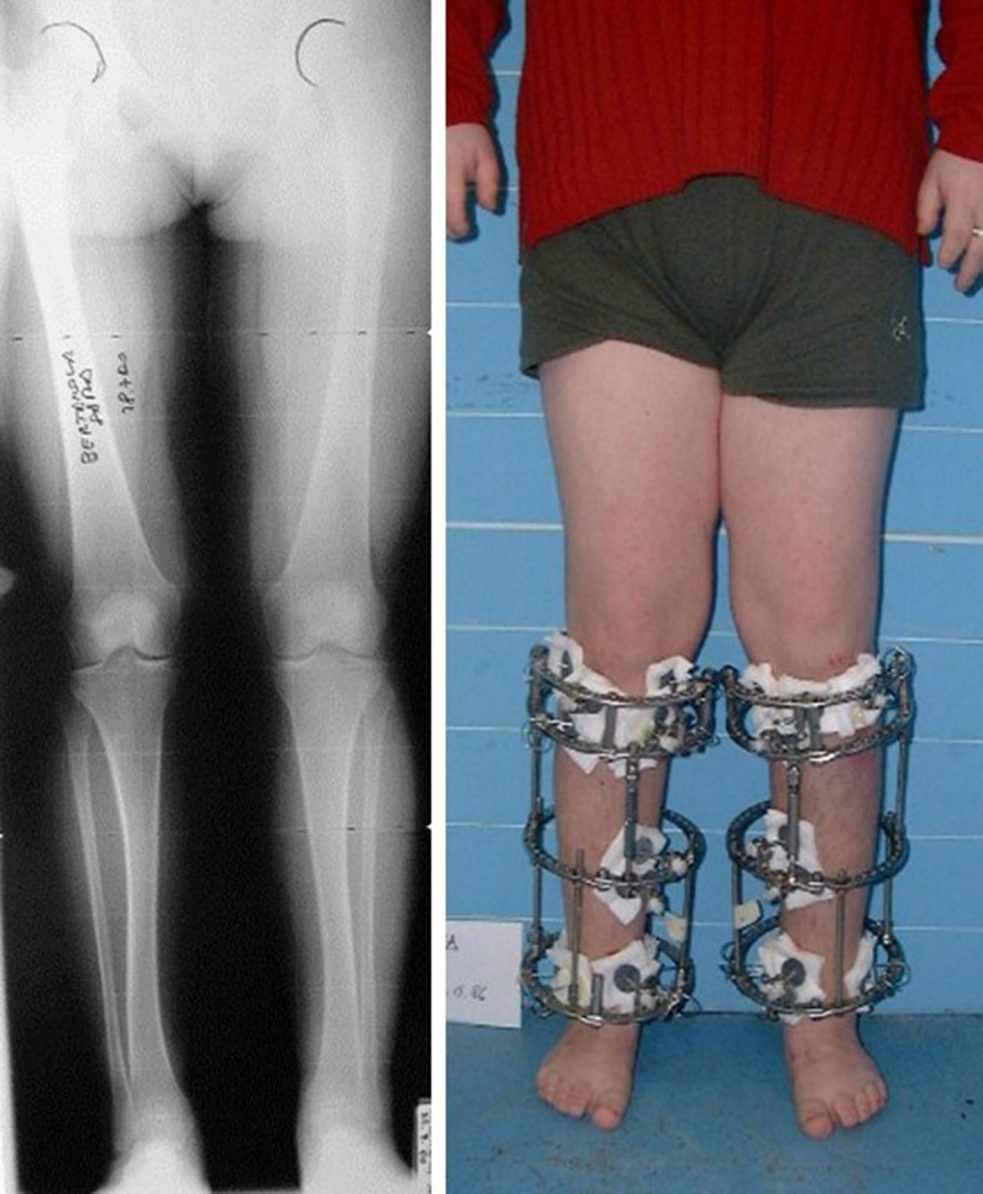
X-rays before treatment in a 17-year-old patient, SD −2
